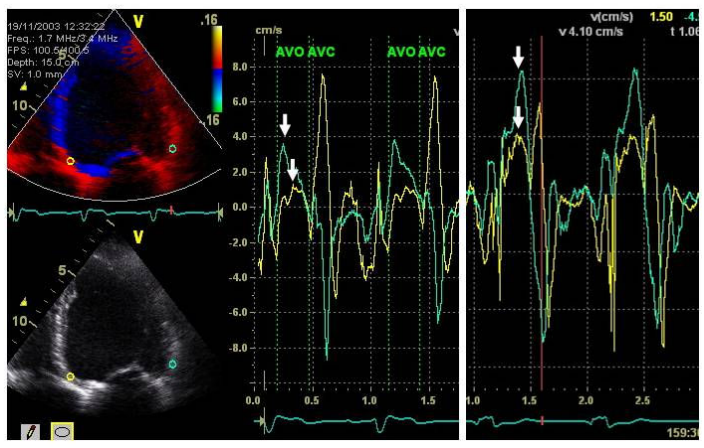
Tissue Doppler Imaging curves with systolic maximal velocity (SMV) preoperatively (*left*) and after implantation of CRT device (*right*), at best VV-interval (sequential pacing LV lead 40 ms before RV lead in this patient), showing improved systolic function, i.e. higher velocity. Signs of less dyssynchrony (arrows) are also seen.
